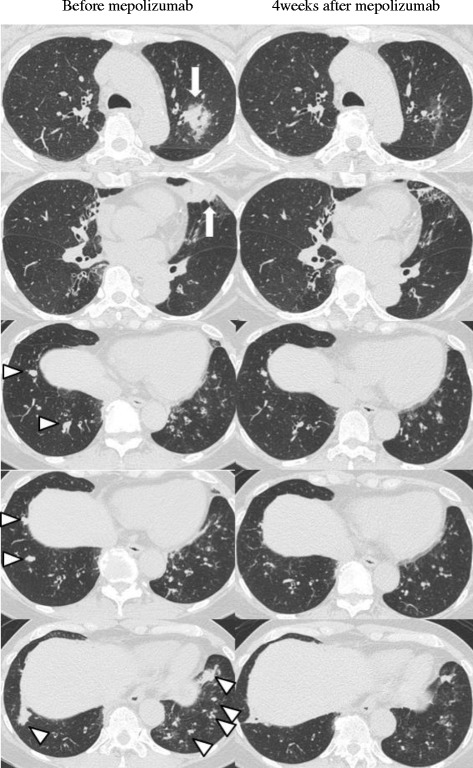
High resolution computed tomography image of the chest showing pulmonary opacities (arrows) in the left upper lobe and mucus plugging (arrow heads) within both lower lobes, prior to mepolizumab treatment. These opacities and mucus plugging were attenuated at 4 weeks after mepolizumab treatment
